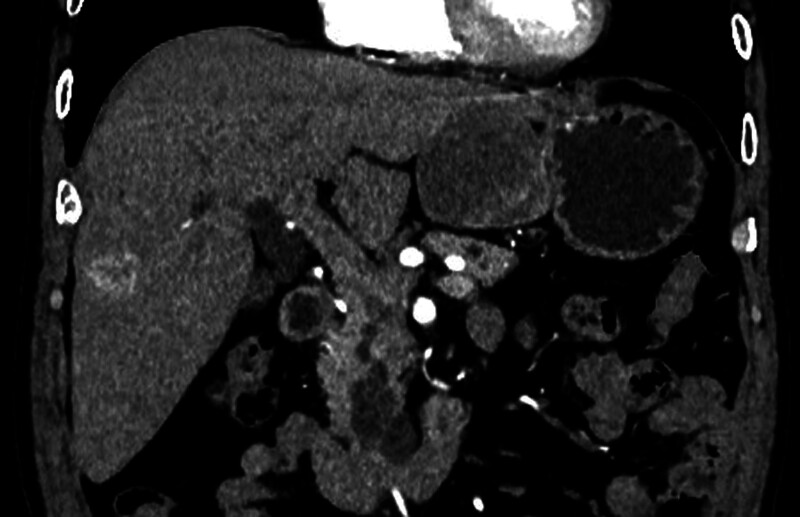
CT shows gastric GIST located in the lesser curvature of the stomach, intrahepatic cholangiocarcinoma located in VIII liver segments and pancreatic IPMN located in the head of the pancreas. CT = computed tomography, GIST = gastrointestinal stromal tumor, IPMN = intraductal papillary mucinous neoplasm.
